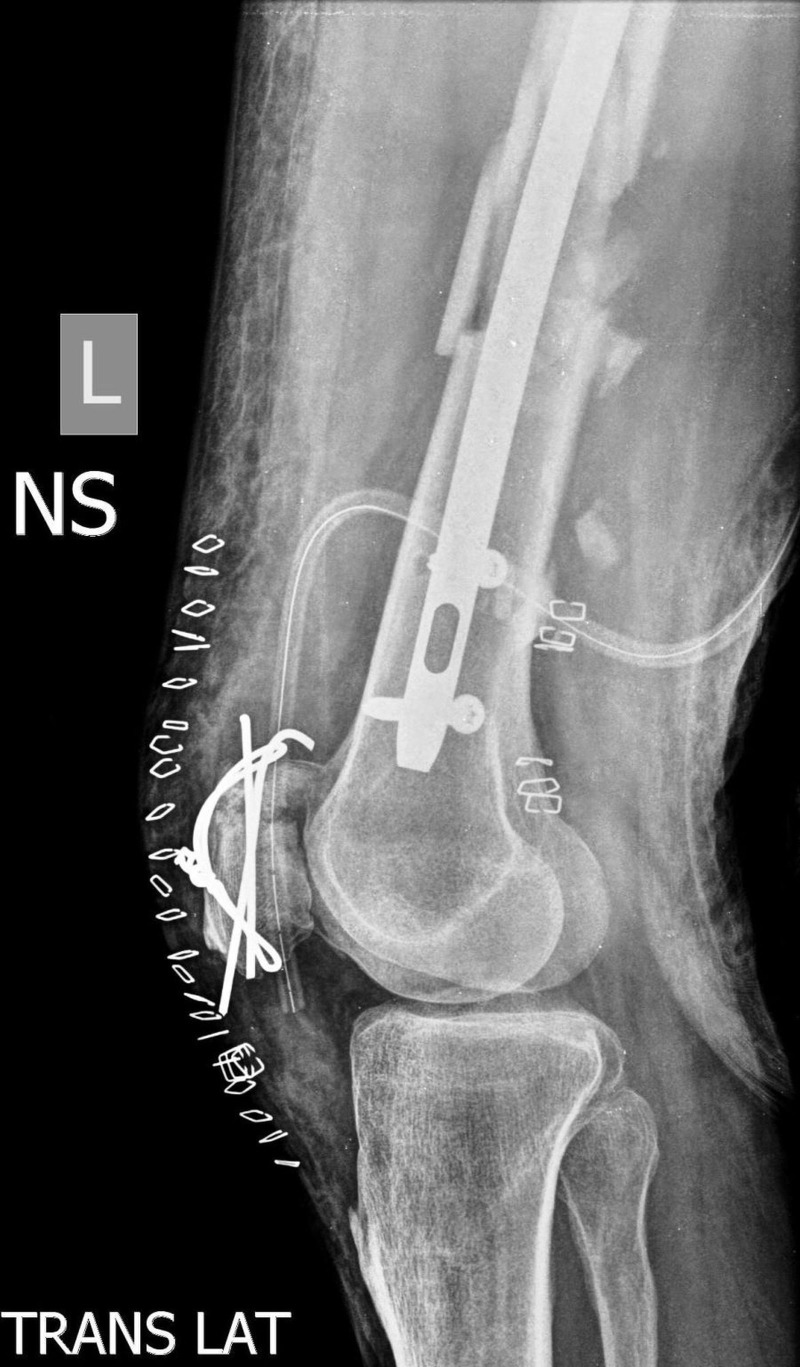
Immediate post-op lateral x-ray of the knee showing patella fracture fixed with tension band wiring
